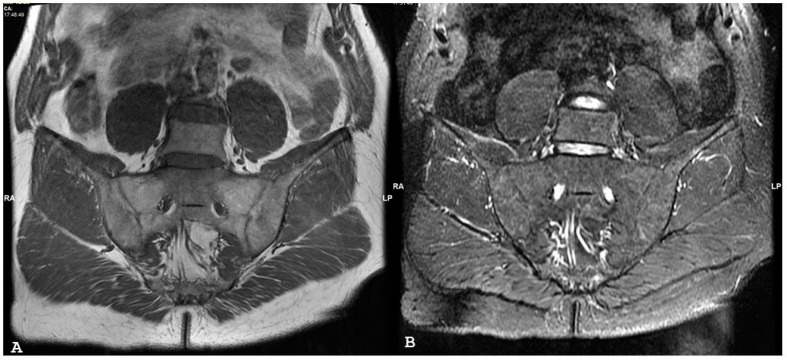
Oblique coronal T1-weighted magnetic resonance imaging (A) demonstrates subchondral fatty replacement and bony fusion consistent with end-stage ankylosis from previous bilateral sacroiliitis. Corresponding oblique coronal short tau inversion recovery sequence (B) shows lack of bone marrow edema.
